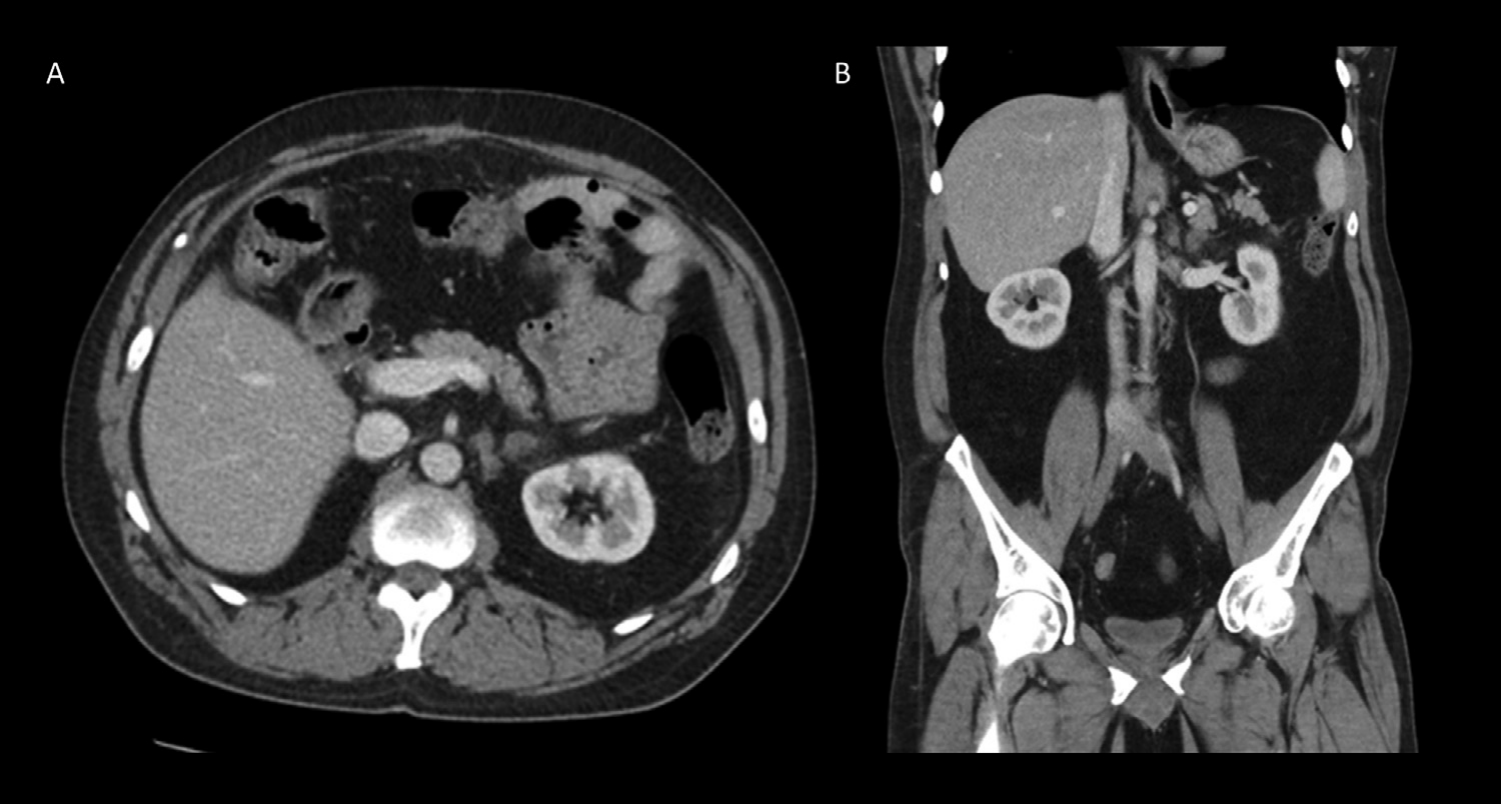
Postsurgical follow up CT, axial (Fig. A) and coronal (Fig. B) demonstrates nodularity at the left adrenal bed likely related to postsurgical change with recommendation for continued surveillance to monitor for recurrence.
